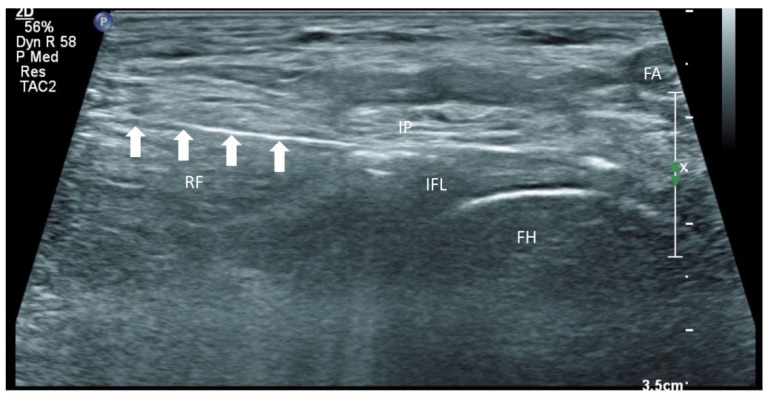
Ultrasound image showing the iliopsoas plane infiltration. FA: femoral artery, FH: femoral head, IP: iliopsoas, RF: rectus femoris, IFL: iliofemoral ligament; white arrow: needle.
